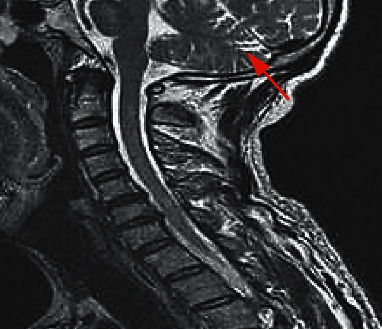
Female NMO patient, 44 years old, with a cross section of the head FLAIR showing a large lesion in the posterior horn of the right ventricle and involving the thalamus.
